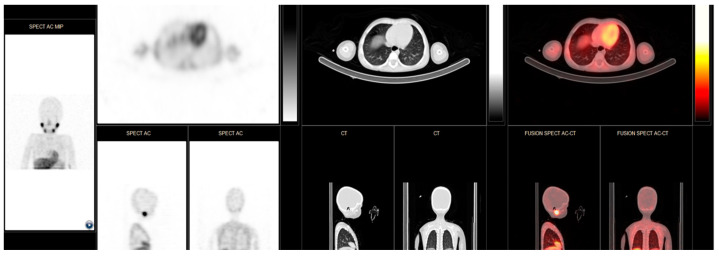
Iodine-123-MIBG SPECT CT images with normal uptake in salivary glands, heart, liver, gastrointestinal tract, and urinary system.
